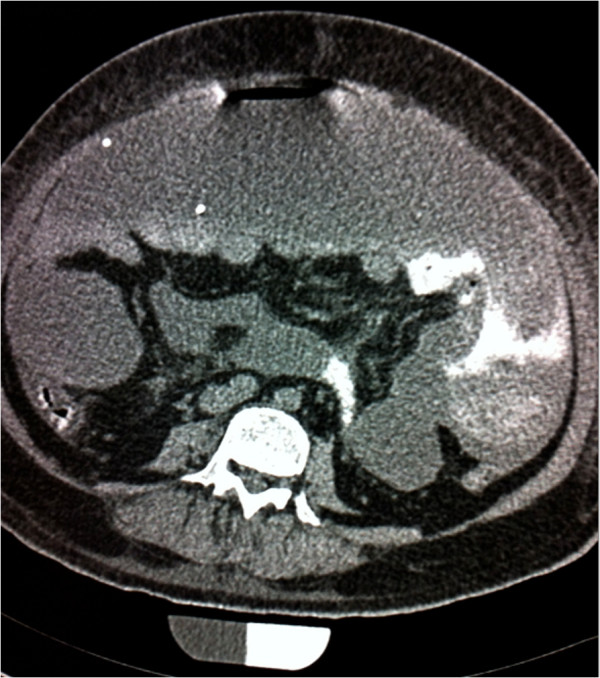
Emergent axial computed tomography scan of the abdomen with contrast enhancement showing extravasation of contrast from the proximal small bowel, indicating bowel perforation.
